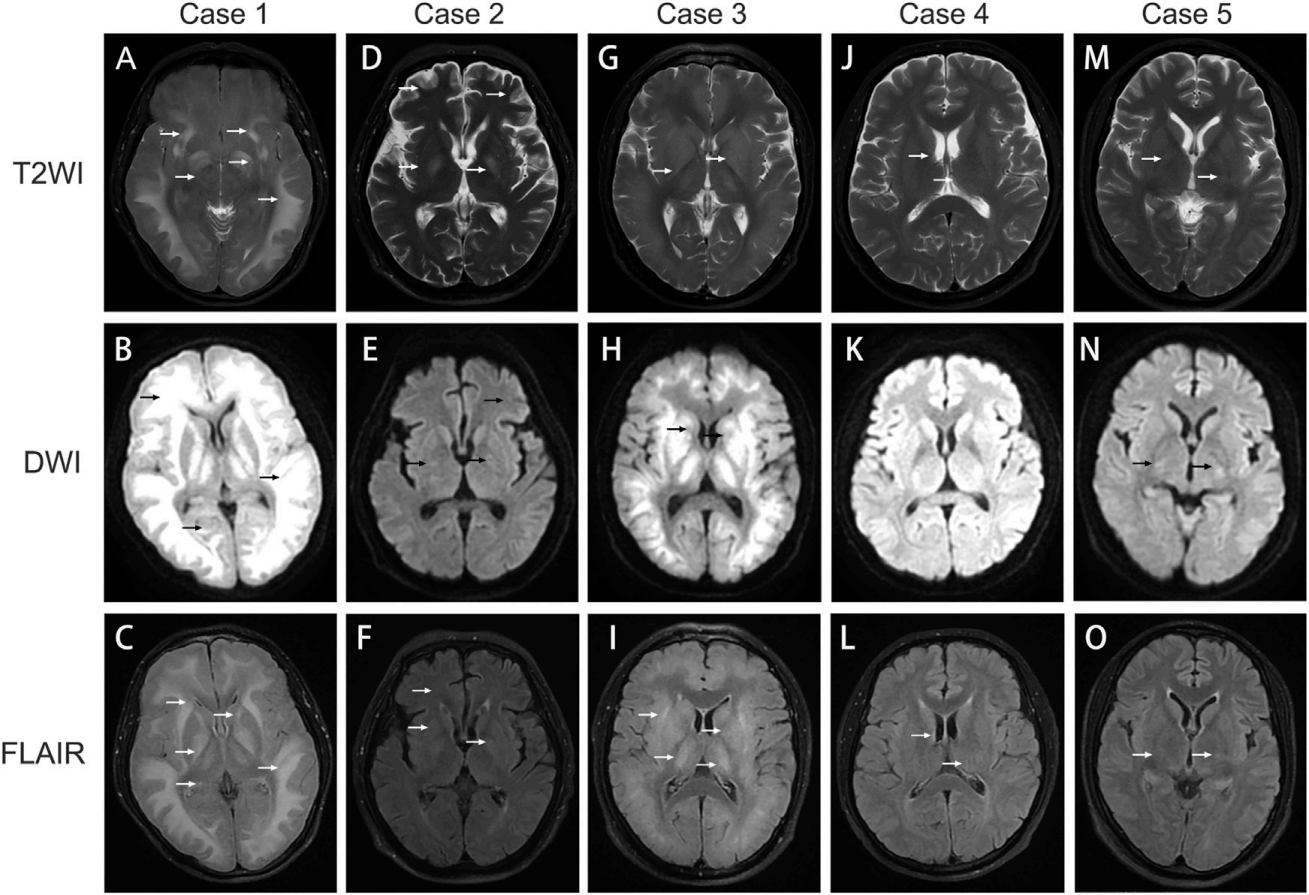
Image findings in 1,2‐DCE induced toxic encephalopathy. (A–C) cerebral MRI images of Case 1; (D–F) cerebral MRI images of Case 2; (G–I): cerebral MRI images of Case 3; (J–L): cerebral MRI images of Case 4; (M–O) cerebral MRI images of Case 5. T2WI: T2‐weighted imaging; DWI: diffusion‐weighted imaging; FLAIR: fluid‐attenuated inversion recovery. The arrows indicate cerebral lesions associated with toxic encephalopathy.
